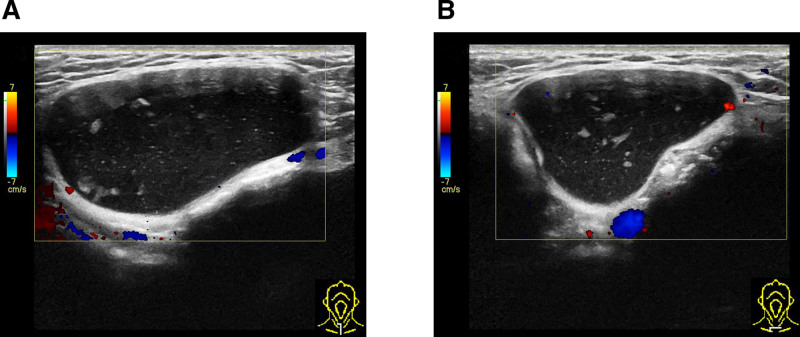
Multiangle ultrasonography revealed a well-circumscribed anechoic cervical mass containing hyperechoic debris. The ultrasound examination was performed using transverse and longitudinal scans in 2 orthogonal directions including craniocaudally (A) and radially (B).
